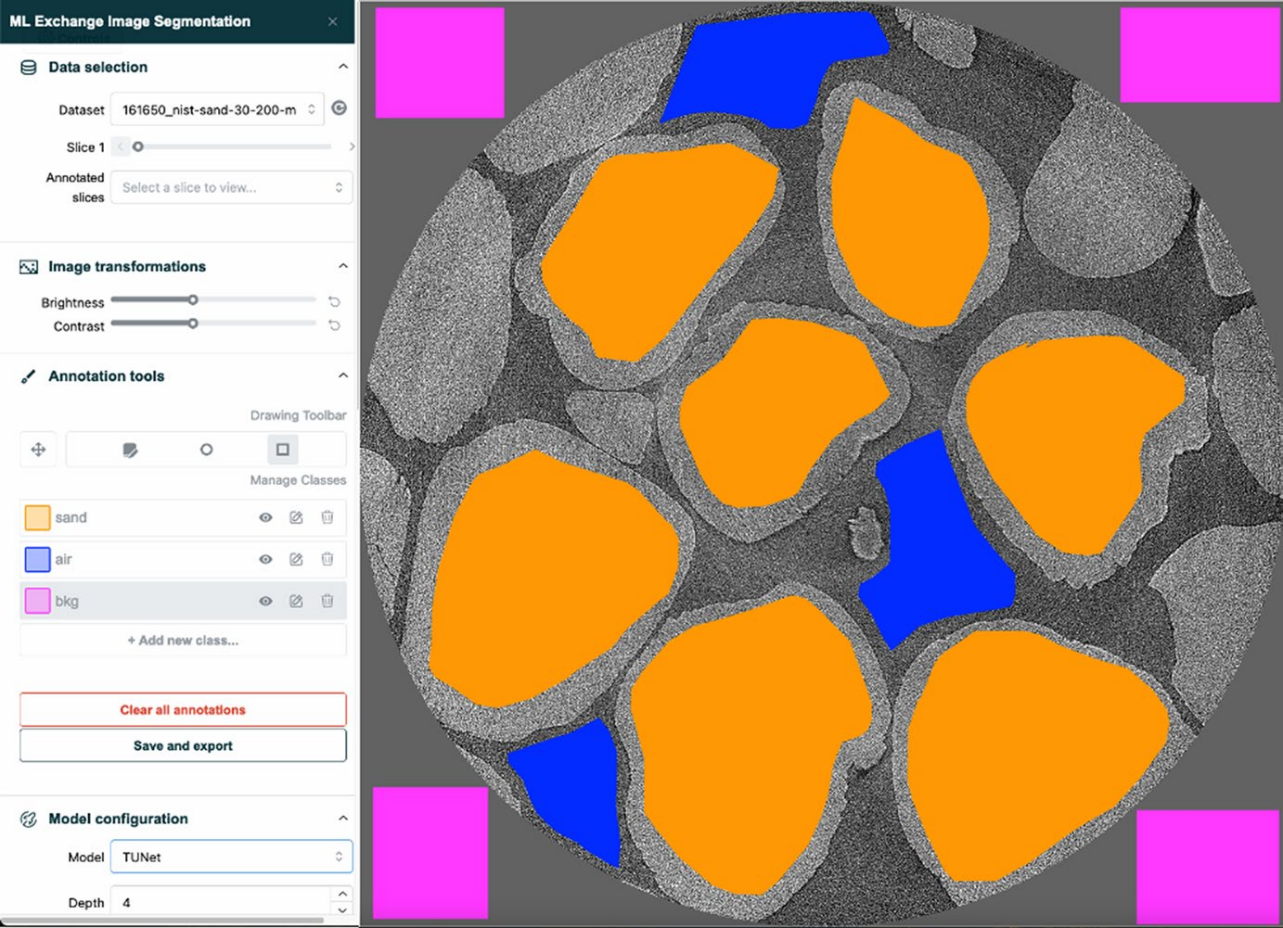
Snapshot of the MLExchange image Segmentation App where a user has created manual segmentation annotations overlaying a selected slice of a X-ray tomography dataset containing sand grains. Aside from creating different annotation classes and shapes, the UI supports navigation of the dataset; basic image transformations with adjustment to brightness, and contrast; loading and saving of created annotations; as well as options to tune the configuration of ML models to be used for training and inference
